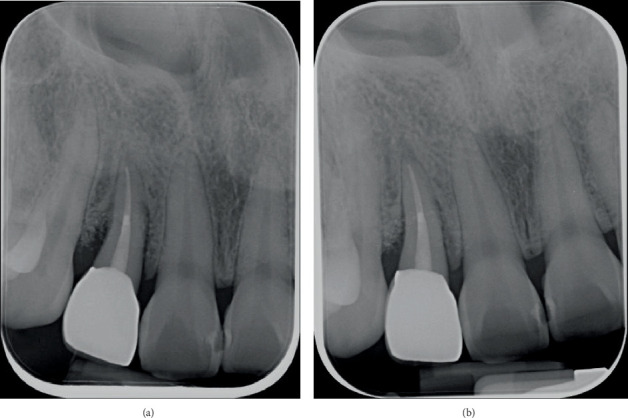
Periapical radiograph of Tooth 12 at (a) 6-month and (b) 1-year review showing bone remodelling in areas of intrabony defect.
